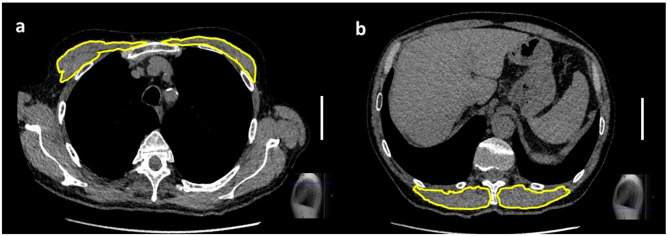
Example of the quantification of muscle area in transverse chest CT scans. (a) Both pectoralis muscles cross sectional areas (CSA) at the level of the aortic vascular outlets are demarked by a yellow line. (b) Delineated erector spinae muscle on the level of the thoracolumbar junction (Th12/L1). The pectoral muscles can be quantified with 15.70 cm^2^ and 11.10 cm^2^ and a mean density of 37.00 HU and 33.20 HU on the right / left side respectively. For the erector spinae the muscle measures 17.00 cm^2^ and 15.20 cm^2^ with a mean density of 32.00 and 41.60 HU on the right / left side respectively. The length of 50 mm is given by the white bar. The slice level is given by a blue line in the lateral scout view displayed in the left part of the image.
